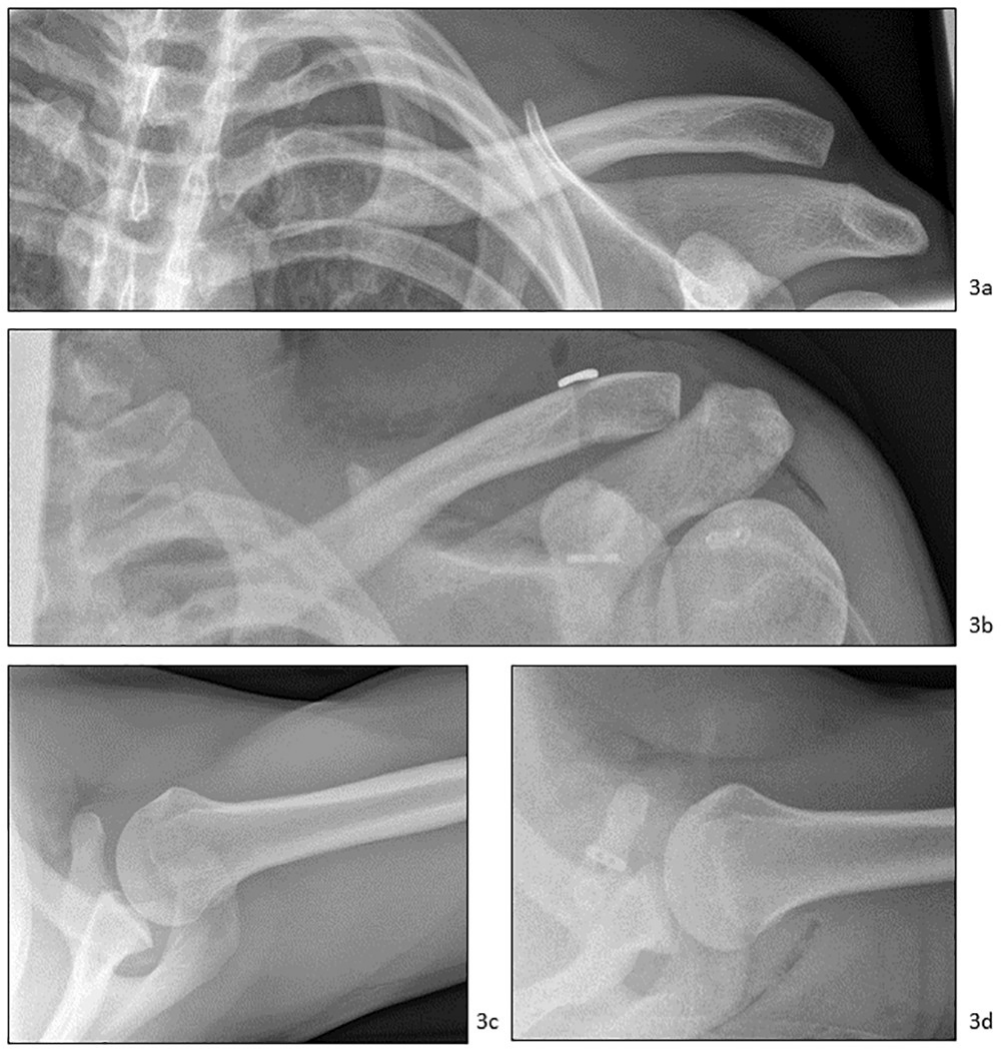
Acromioclavicular joint dislocation treated with Tight Rope^®^ stabilization. Preoperative and postoperative x-rays of an acromioclavicular joint (ACJ) dislocation type Rockwood III with horizontal instability treated with Tight Rope^®^ stabilization (15-year-old boy, fall while playing soccer). a) Preoperative anterior-posterior view. b) Postoperative anterior-posterior view. c) Preoperative axial view. d) Postoperative axial view.
